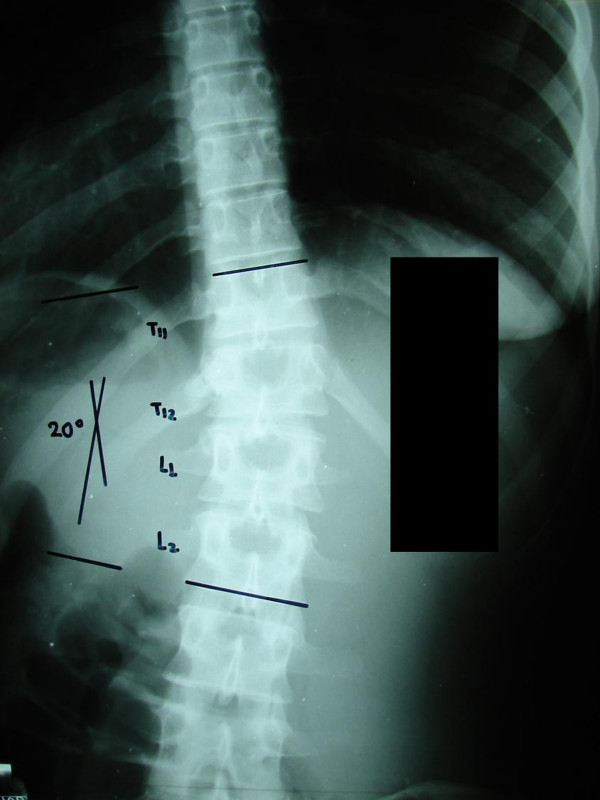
Postero-anterior radiograph at age 21. The tumour is indiscernible.
